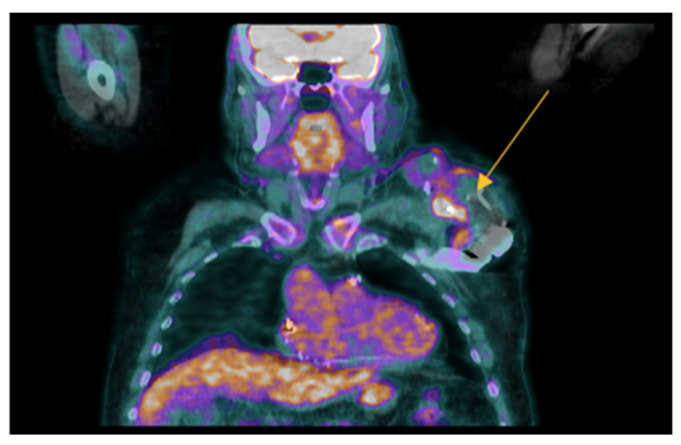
Pacemaker pocket infection. In this case, we reported an image of a 18F-FDG PET/CT scan showing intense uptake at the patient’s pacemaker pocket (yellow arrow) (male, 76 years old).
